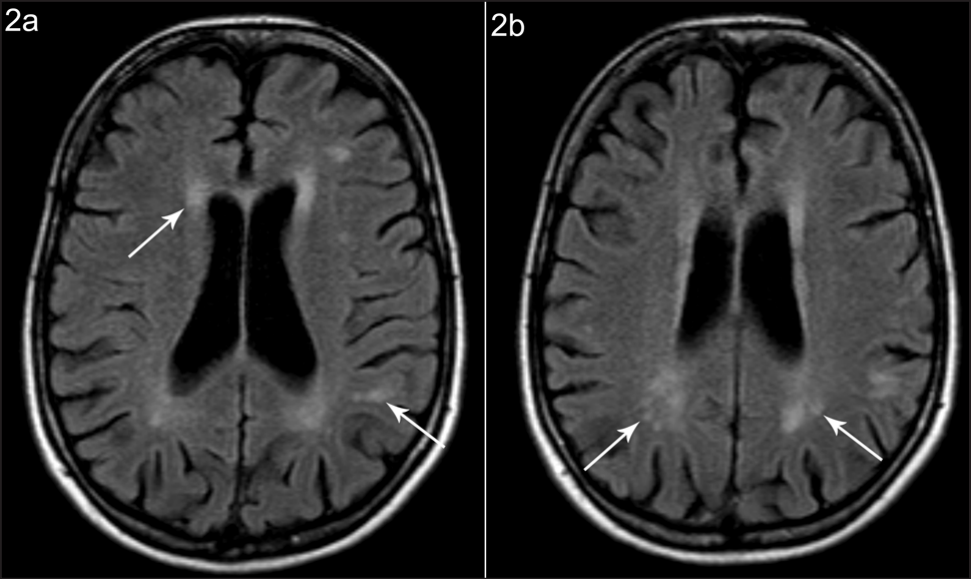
Patient A, axial FLAIR weighted images at different sections show (a) periventricular white matter hyperintensity and multiple hyperintense lesions in the subcortical white matter, with partial confluence and (b) white matter hyperintensity posterior and superior to the trigone.
